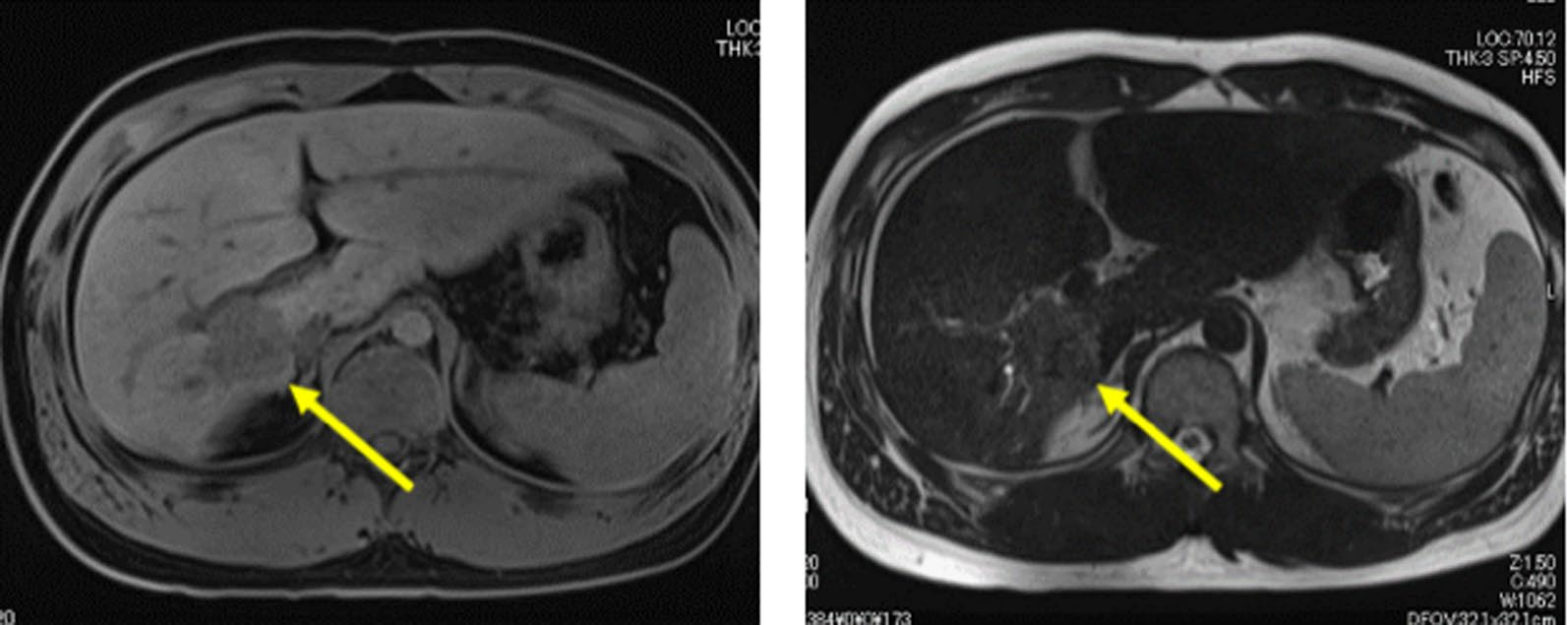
Magnetic resonance imaging (MRI) showing low signal tumor on T1-weighted image (T1WI), high signal marginal region on T2-weighted images (T2WI) and high signal marginal region on diffusion weighted image
